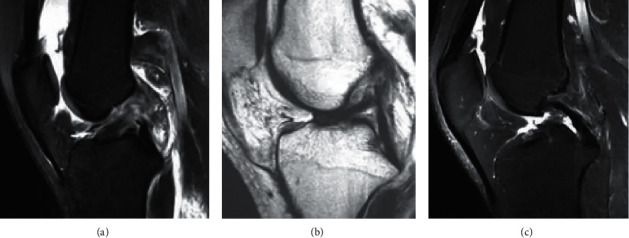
MRI images of the patients. (a) ACL discontinuity (49-year-old female patient), (b) ACL drooping sign (58-year-old male patient), and (c) ACL disappearance (55-year-old male patient).
